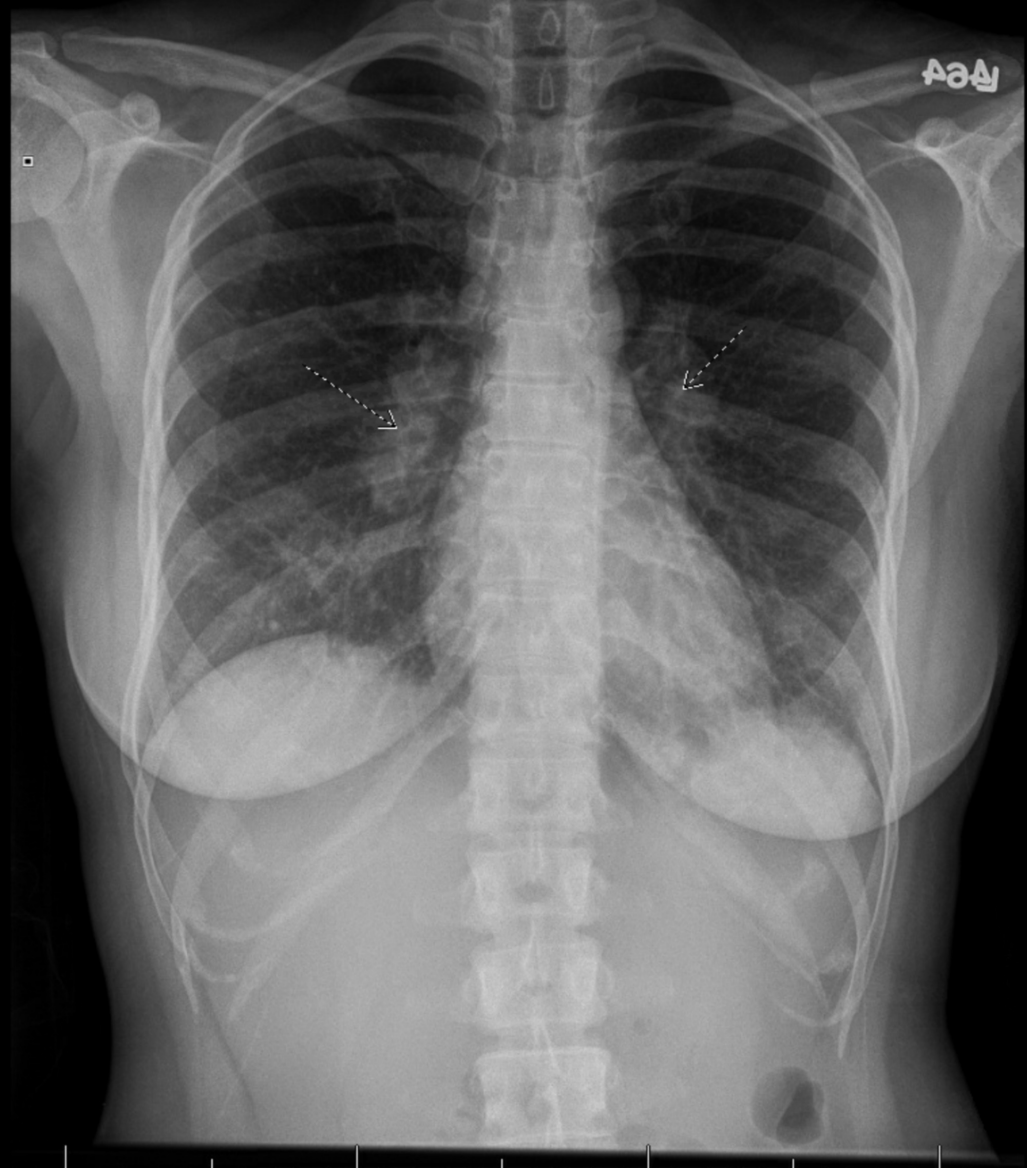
Chest x-ray showing bilateral hilar lymphadenopathy
